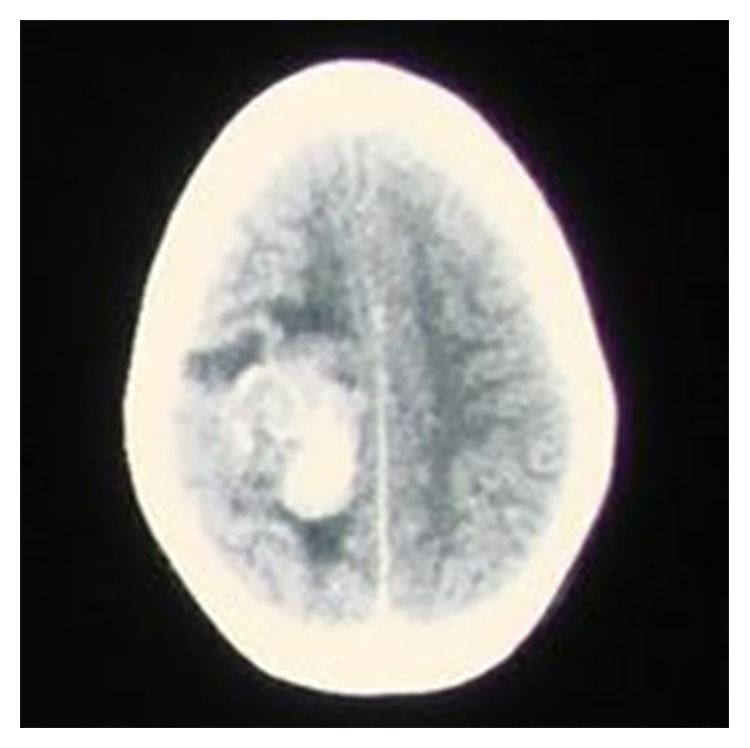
Preoperative CT scan brain showing a calcified lesion in the right temporoparietal region.
